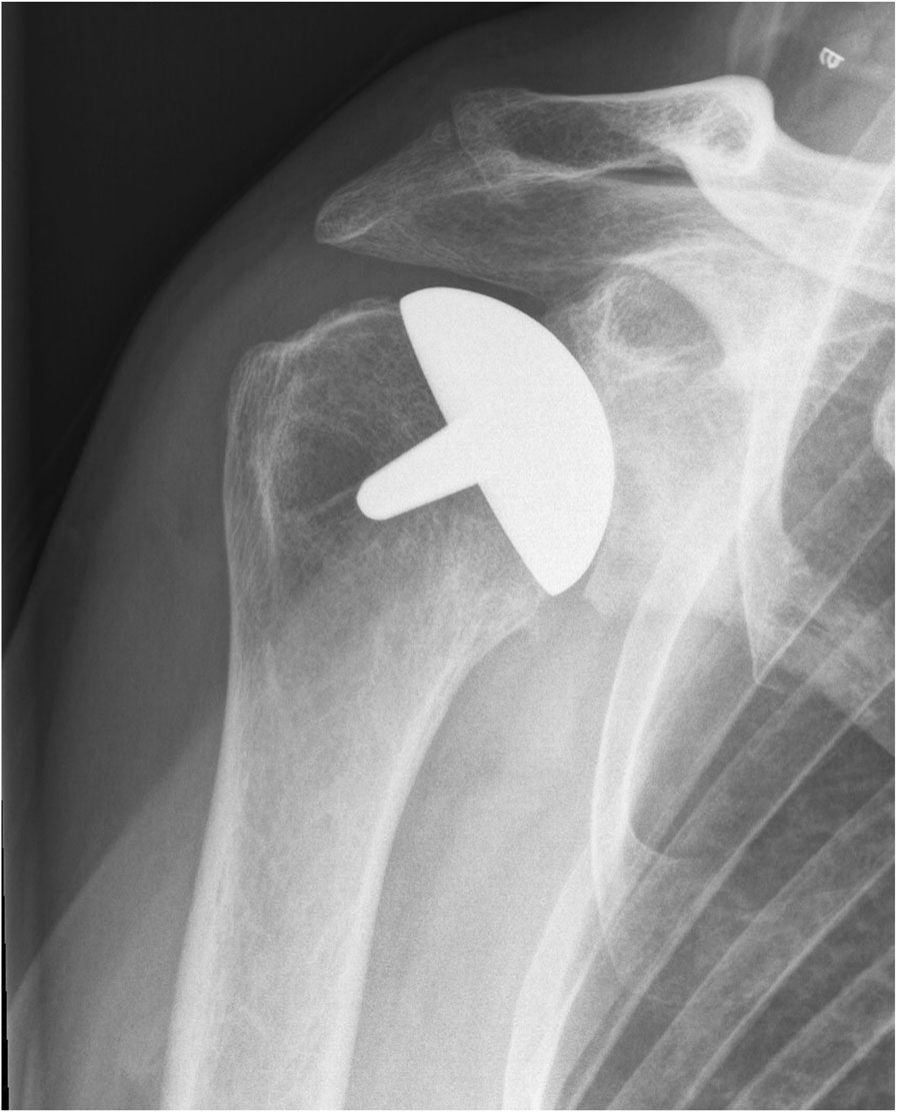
Post-implant AP radiograph where all observers classified the size, inclination, and stuffing of the joint as anatomical
